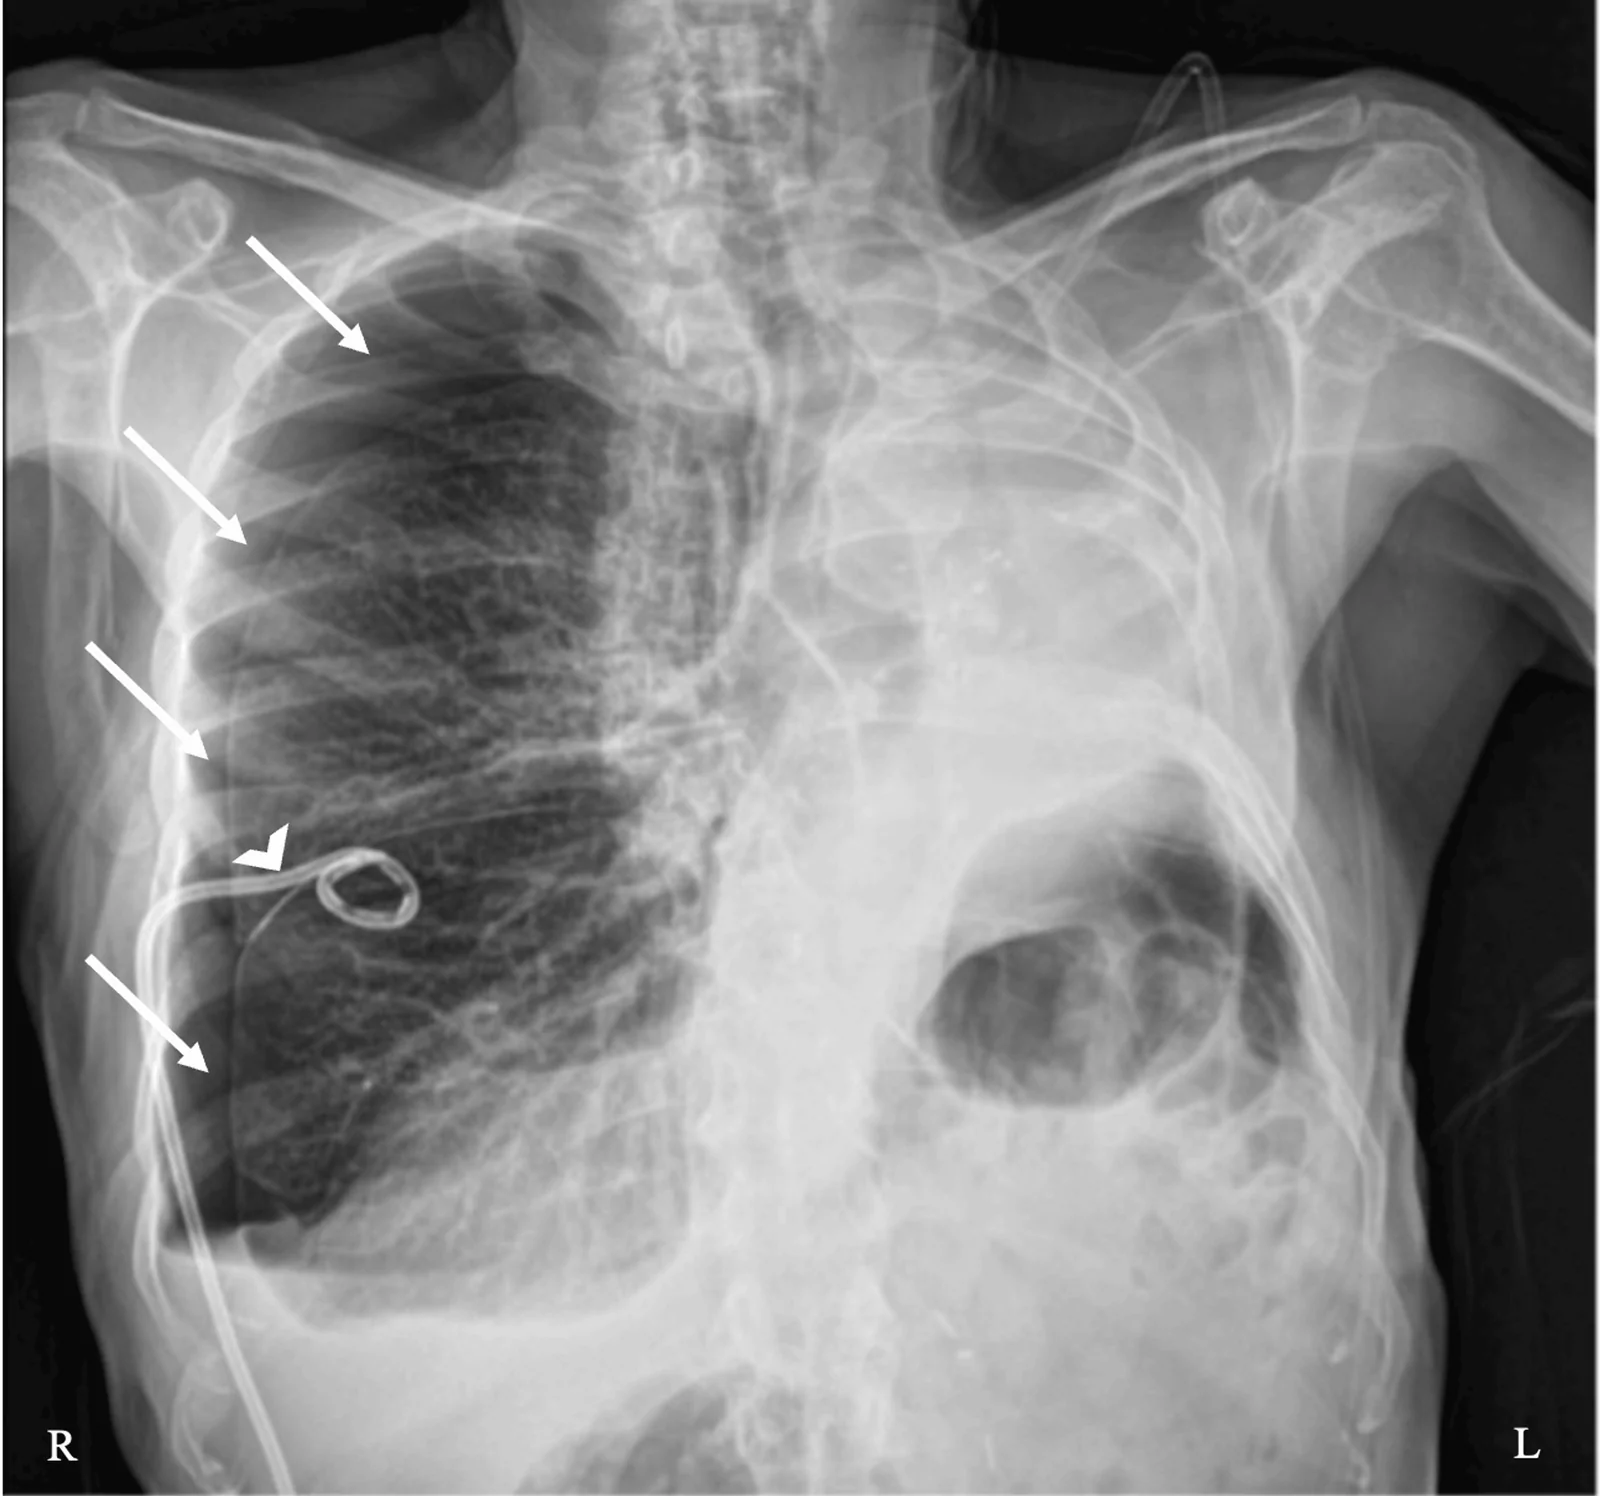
Preoperative chest radiograph Chest radiograph obtained 12 days before surgery, demonstrating recurrent right pneumothorax (arrows), right-sided chest tube (arrowhead), and expected post-pneumonectomy changes (opacification of the left hemithorax, leftward mediastinal shift, and elevation of the left hemidiaphragm). L: left; R: right
